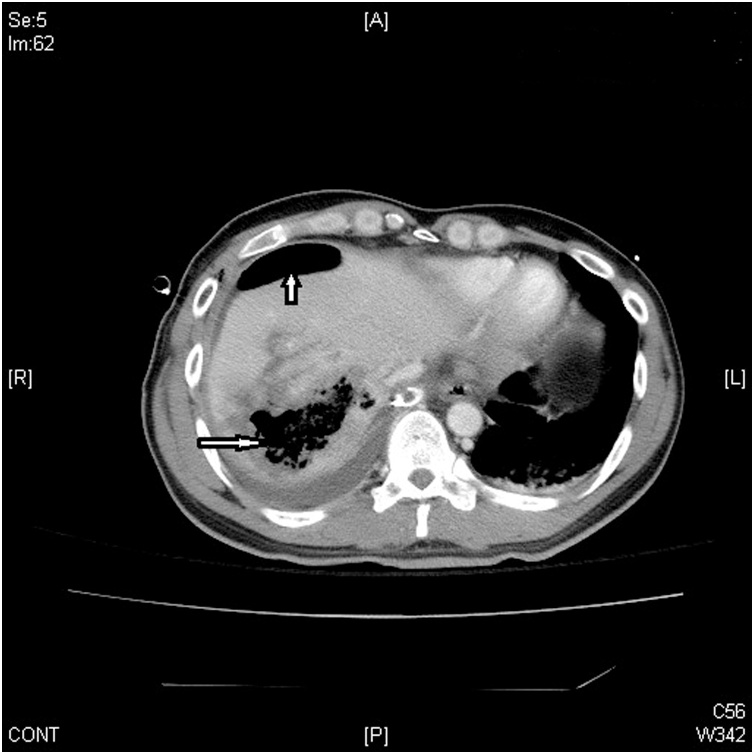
Abdominal computed tomography scan of the same patient performed 4 h after chest radiography, showing a gas-containing liver abscess over the previous injured liver (long arrow). The perihepatic fluid accumulation and intra-peritoneal free air indicated rupture of abscess (short arrow).
